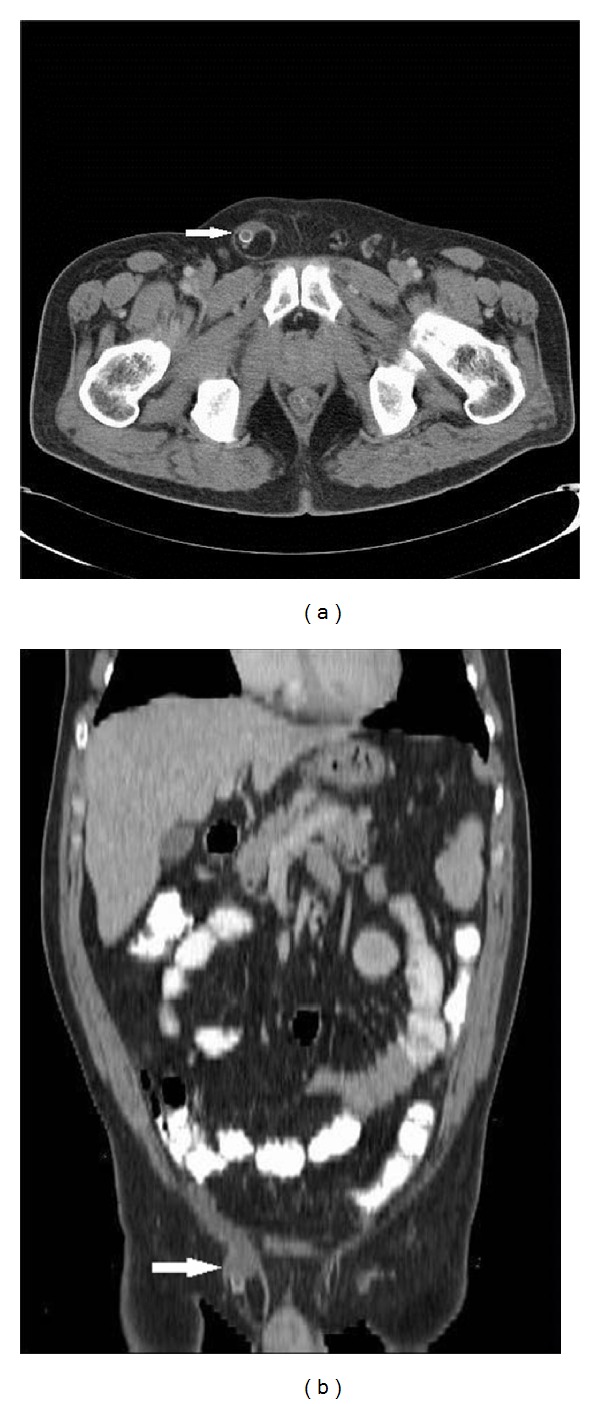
Amyand's hernia was showed after intravenous (IV) contrast CT of the abdomen. Axial (a) and coronal MPR (b) images. A small amount of fluid around appendix with appendiceal fecalith is seen on the axial image (arrow). The inflamed appendix is particularly well, as seen on the coronal reformats (arrow).
